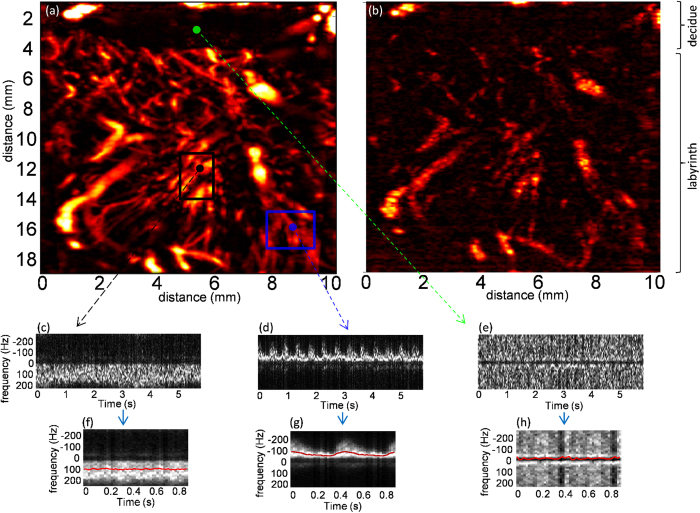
Ultrafast imaging of the placenta. (a,b) Respectively UDF and conventional Power Doppler image of the placenta containing maternal and fetal blood. (c–e) UFD PW Doppler of respectively a non pulsatile vessel, a pulsatile vessel and noise within one spatial pixel. (f–h) Averaged UFD PW Doppler of respectively a non pulsatile vessel, a pulsatile vessel and noise within one spatial pixel.
